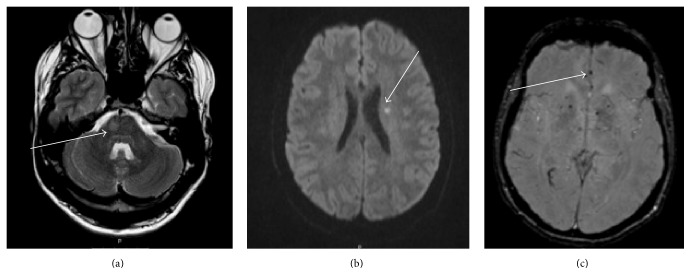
MRI scan of brain. MRI of brain. Axial T2-weighted image showing T2 hyperintense lesion within the right pons (arrow) (a). Axial DWI (B1000) demonstrates restricted diffusion within the left centrum semiovale (arrow) (b). Gradient recalled ECHO T2 susceptibility weighted imaging showing diffuse hypointense lesions suggestive of microhaemorrhages (arrow) (c).
